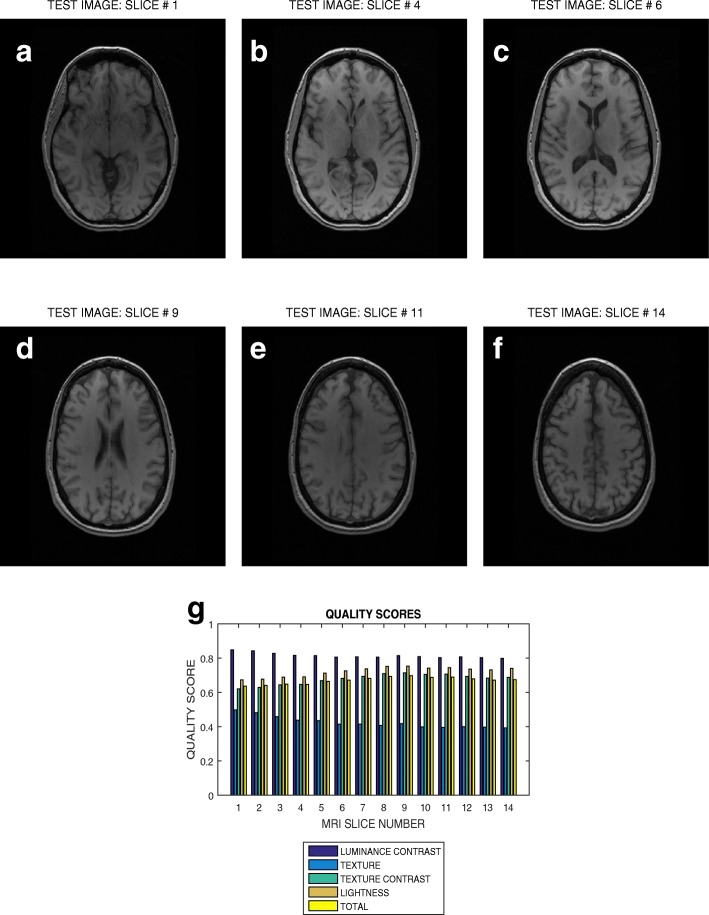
Six slices with indices (a) 1, (b) 4, (c) 6, (d) 9, (e) 11 and (f) 14 in a T1 weighted MRI volume data from NeuroRx Research Inc., (g) luminance contrast, texture, texture contrast, lightness and total quality scores of 14 successive slices in the MRI volume data
